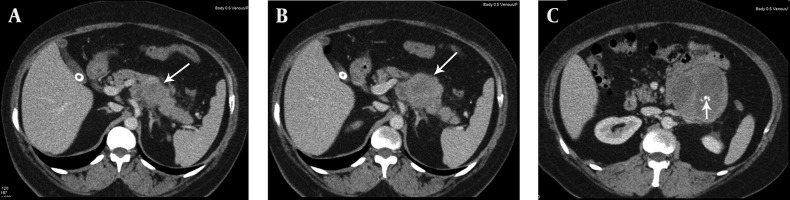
A, B and C) Contiguous portal venous phase axial contrast enhanced CT images show a large heterogeneous mass with central necrotic areas and calcifications (short arrow) arising from the tail of the pancreas (long arrows) extending near the anterior of the left kidney and aorta.
